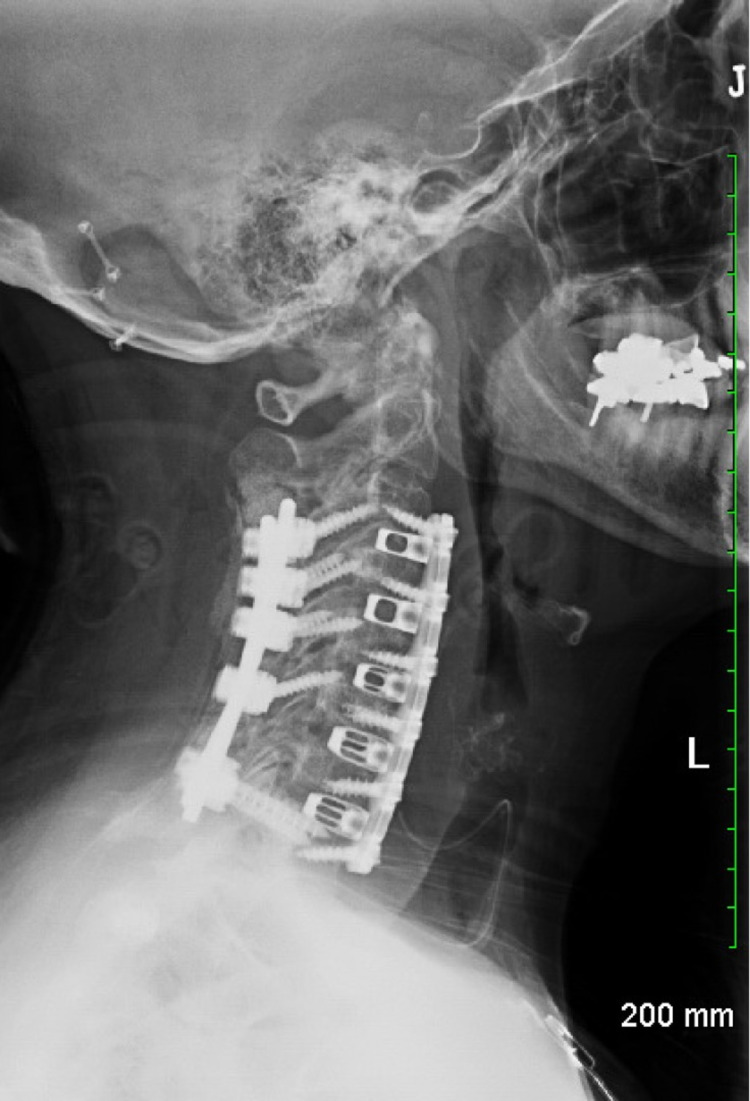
Lateral X-ray of the cervical spine Proper placement of all hardware
